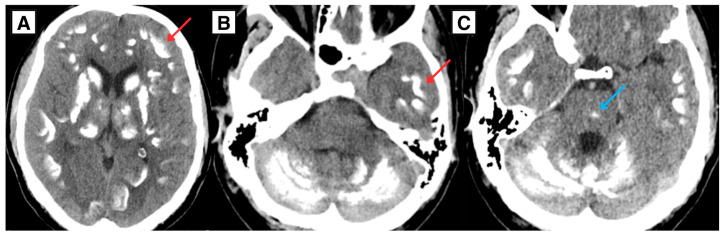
Non-contrast axial brain CT demonstrates extensive intracranial calcifications with a basal ganglia predominant distribution, consistent with Fahr-like intracranial calcifications. (A) Symmetric calcifications in the basal ganglia and thalami. (B) Extensive calcifications in the subcortical white matter and cerebellar structures. (C) Calcification involving the midbrain. Red arrow: symmetric subcortical white matter calcifications. Blue arrow: midbrain calcification.
